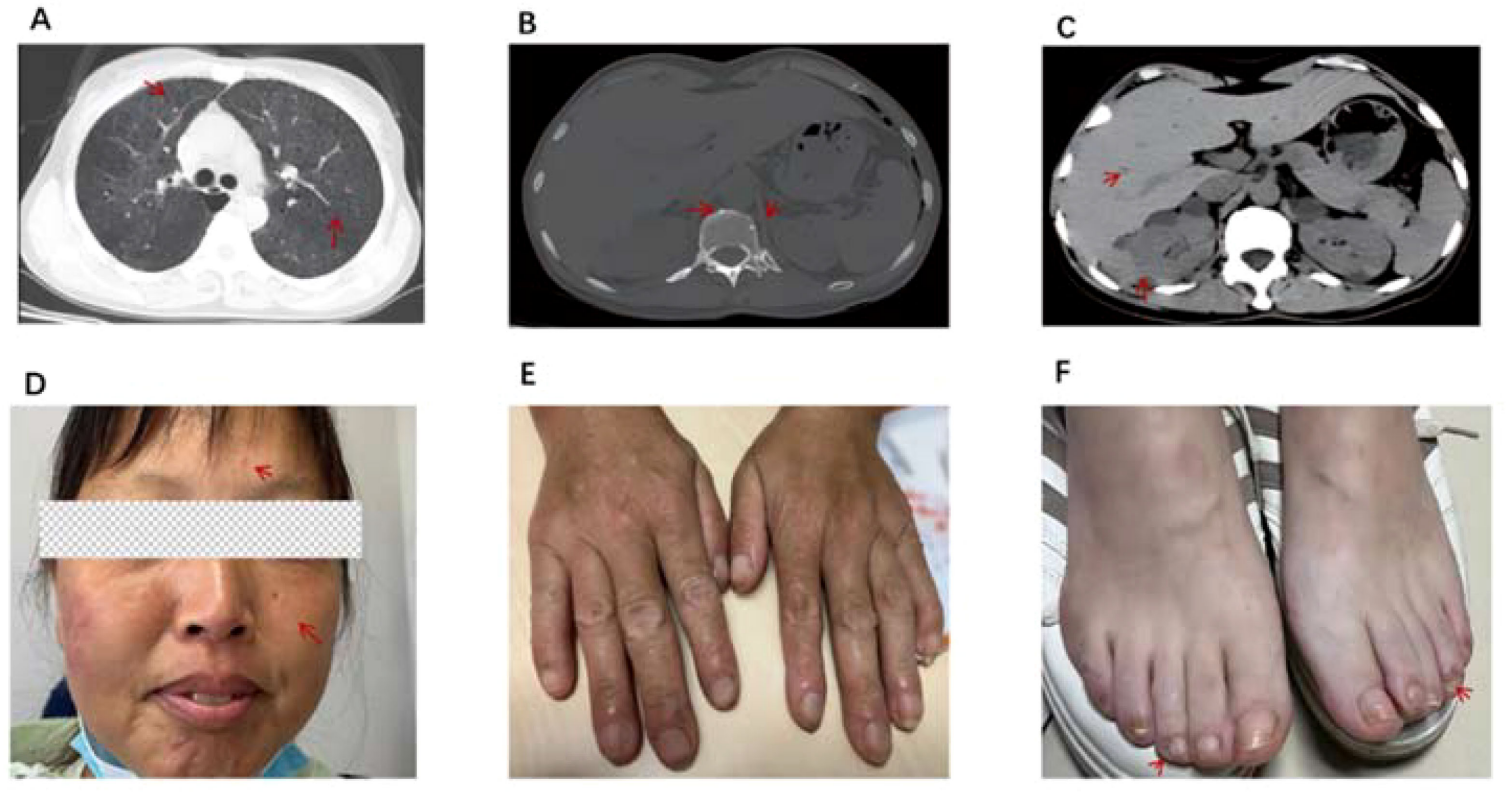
(A) CT scan of the chest shows pulmonary cystic degeneration is the same as before, however, ground-glass changes in the lungs are less severe than before. (B) CT of the spine shows vertebral osteosclerotic nodules remain. (C) CT scan of the abdomen showed multiple nodules in the liver and multiple high-density nodules in the kidneys. (D) Fibroids on the forehead and face are smaller than before. (E, F) Periungual fibroids are partially reduced. The red arrows indicate the abnormalities observed on imaging and the patient's abnormal signs after treatment.
